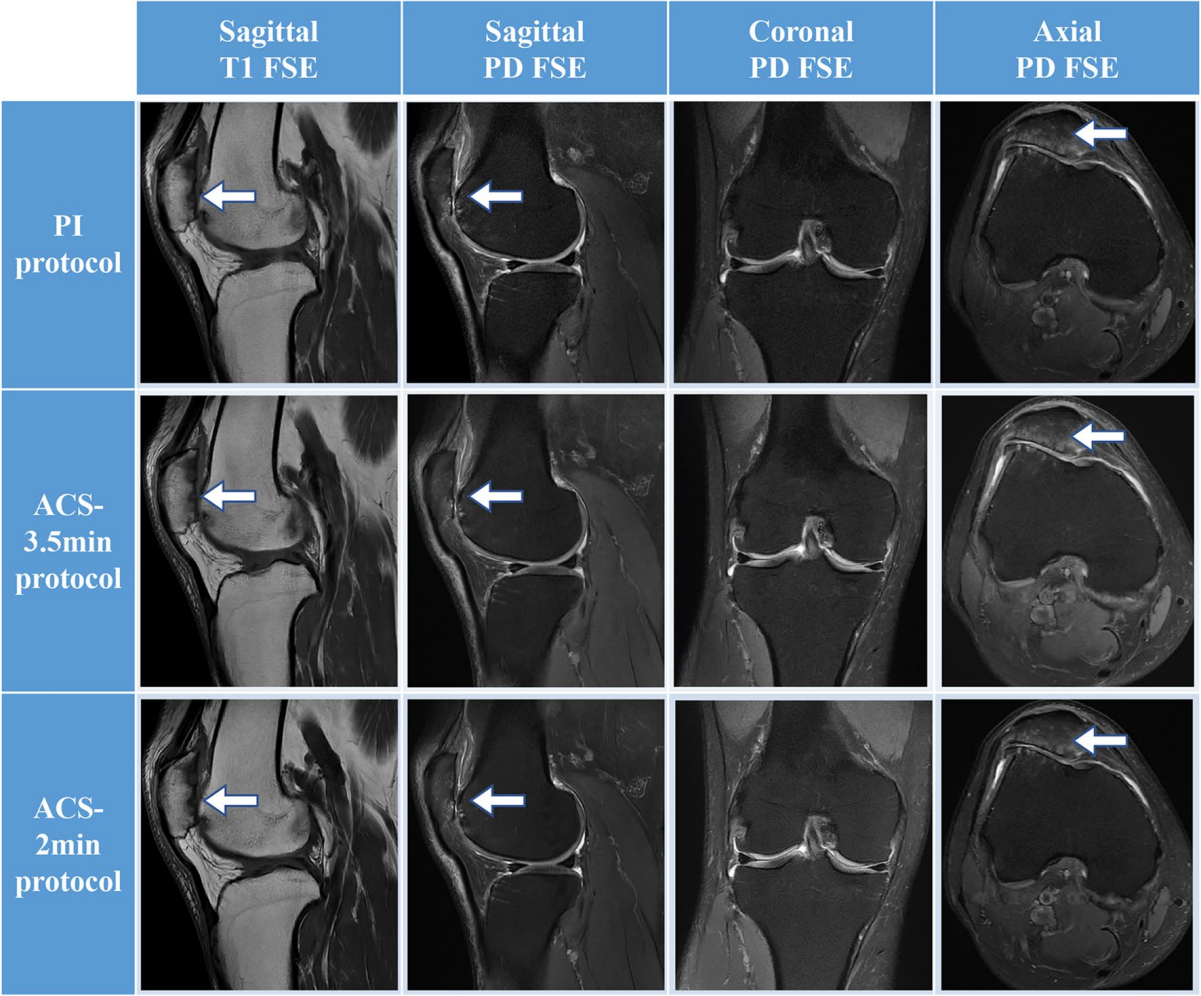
MRI images of a 44-year-old male athlete with patellofemoral arthropathy. From left to right, sagittal T1-weighted images, proton density–weighted fat-suppressed (PDFS) sagittal, coronal, and axial images of fast spin-echo (FSE) imaging, respectively. Patellofemoral and femoral talocrural articular cartilage and subchondral bone damage (arrows) are clearly demonstrated on sagittal T1, sagittal, and transverse PDFS images. The meniscus is intact on coronal PDFS images
